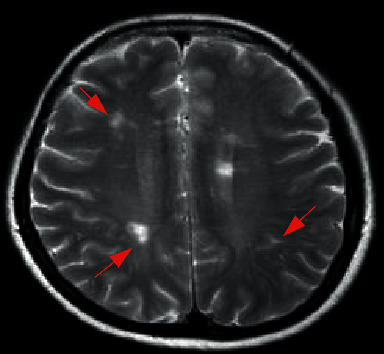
Female NMO patient, 60 years old. FLAIR cross section of the head shows multiple nonspecific focal lesions under the cerebral cortex.
